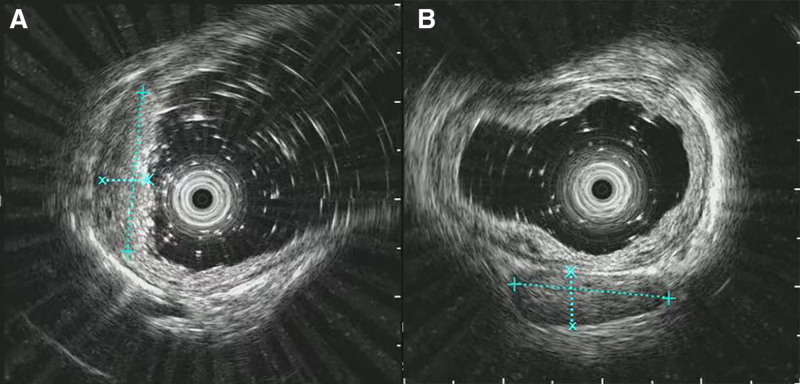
Endoscopic ultrasound findings. (A) Marked thickening of the mucosal layer; (B) enlarged lymph node detected in the periesophageal mediastinum.
